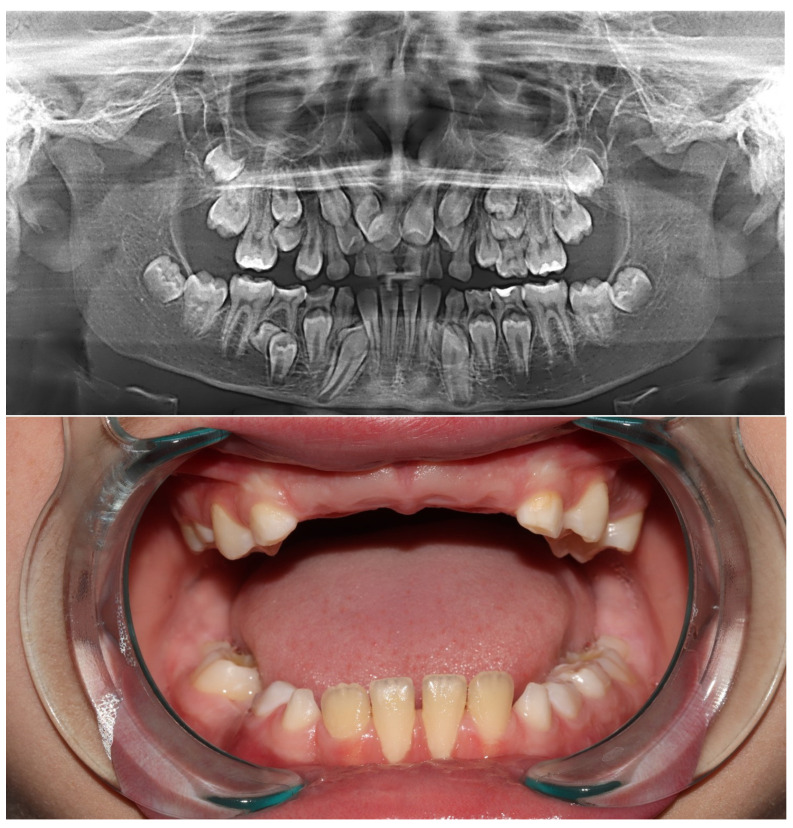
The patient was admitted to the Dental Clinic of the Faculty of Medical Sciences, University of Kragujevac due to unerupting teeth in the frontal region. His parents reported an unremarkable medical history. During the general physical examination of the hands and the phenomenon of shoulders close to the front of the body, it did not indicate that it was Cleidocranial Dysplasia (CCD). However, a molecular genetic analysis was proposed to exclude a diagnosis of CCD. Considering the patient’s age (eleven years), a retroalveolar X-ray was initially performed, followed by a panoramic X-ray. After a thorough examination of the panoramic X-ray, primary teeth were extracted in the frontal region, and it was decided that a CBCT scan would be performed to obtain more data regarding the supernumerary teeth.
